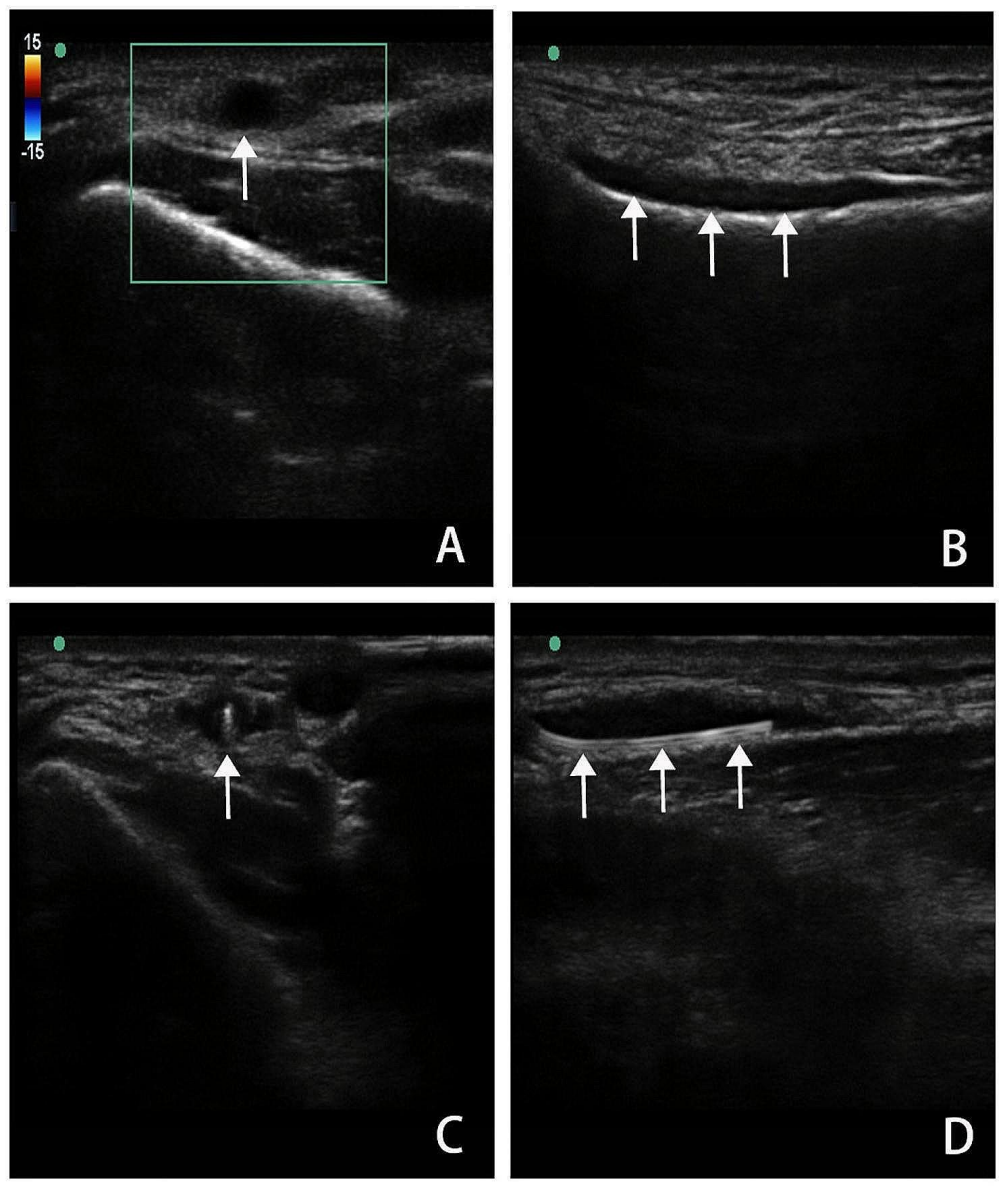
Ultrasound images showing transverse (A, ↑ black circle was radial artery) and long-axis (B, ↑ black fusiform was radial artery) of the radial artery with RAO. The radial artery with the echogenic needle tip within the lumen in transverse (C, ↑ white dot in the round black was needle tip). The movable guide wire was located in the true lumen through ultrasound (D, ↑ white strip was movable guide wire)
